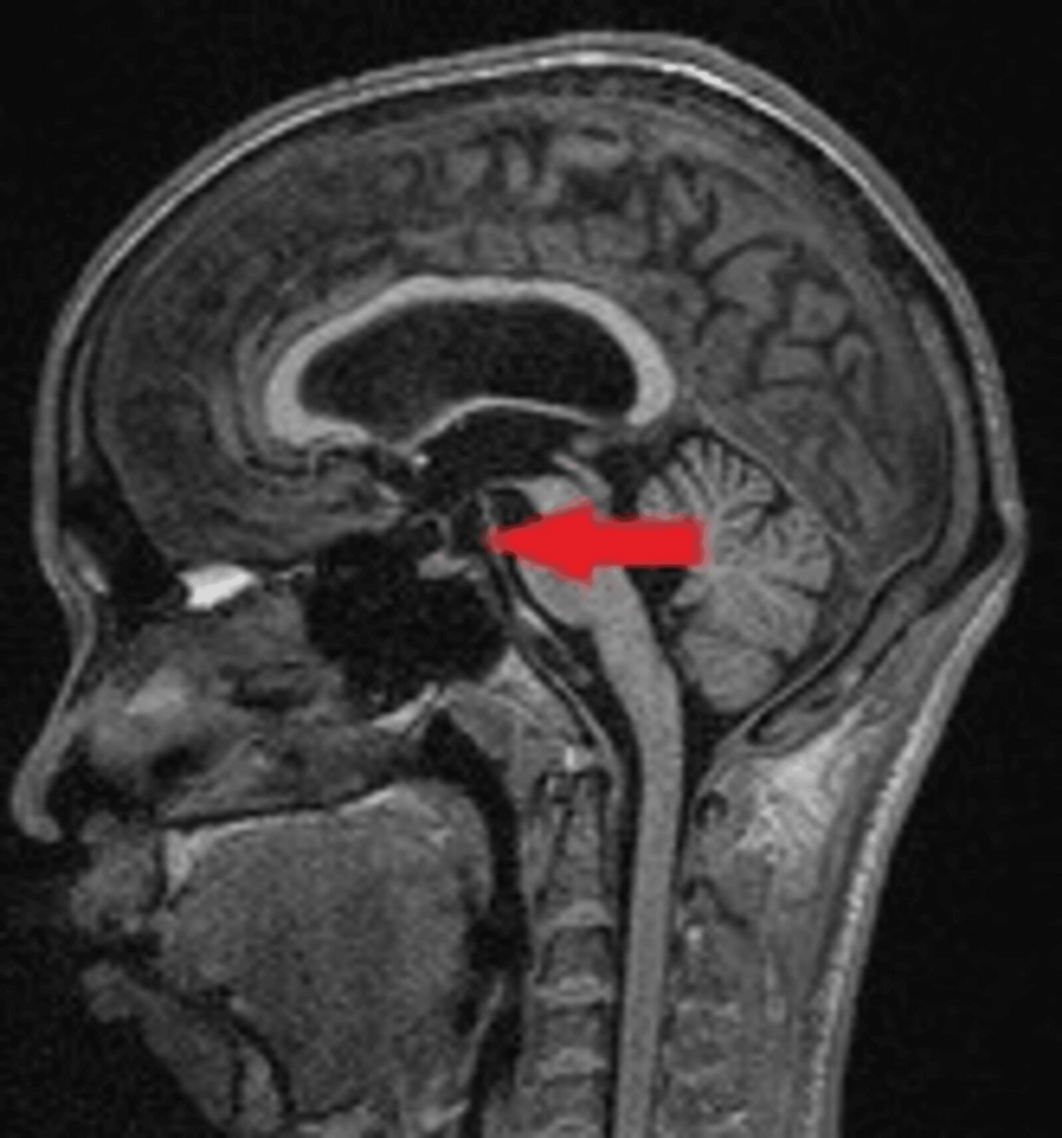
MRI of the brain in T1 MPRAGE sagittal view showing absent posterior pituitary bright spot (red arrow). MRI: Magnetic resonance imaging; MPRAGE: Magnetization prepared rapid gradient echo
